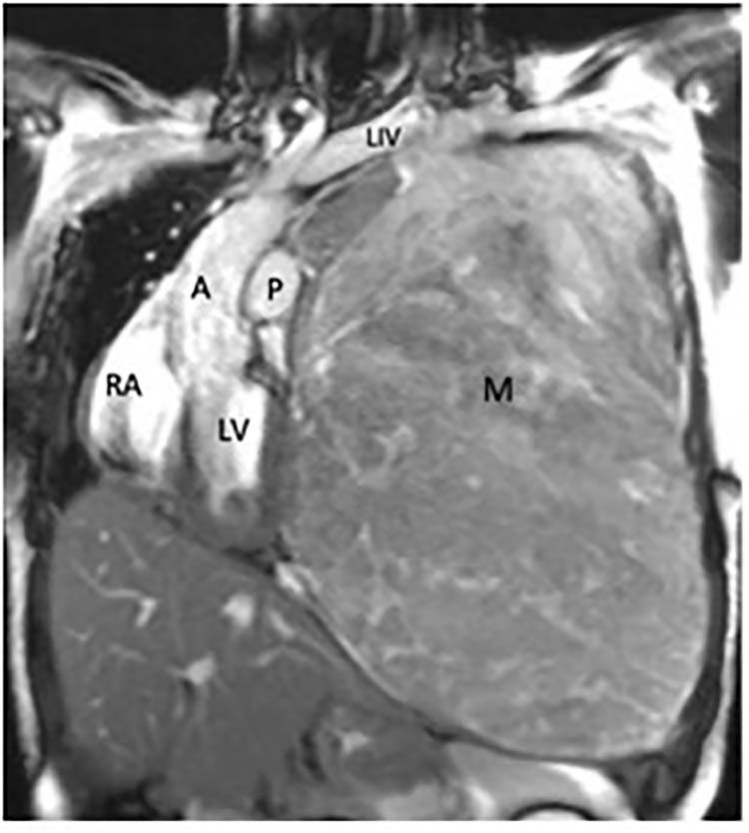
Coronal magnetic resonance image, showing the large heterogeneous mass occupying the left hemithorax. Note severe displacement of the heart and vasculature as well as complete atelectasis of the lung (L), left ventricle (LV), right atrium (RA), aorta (A), pulmonary trunk (P).
